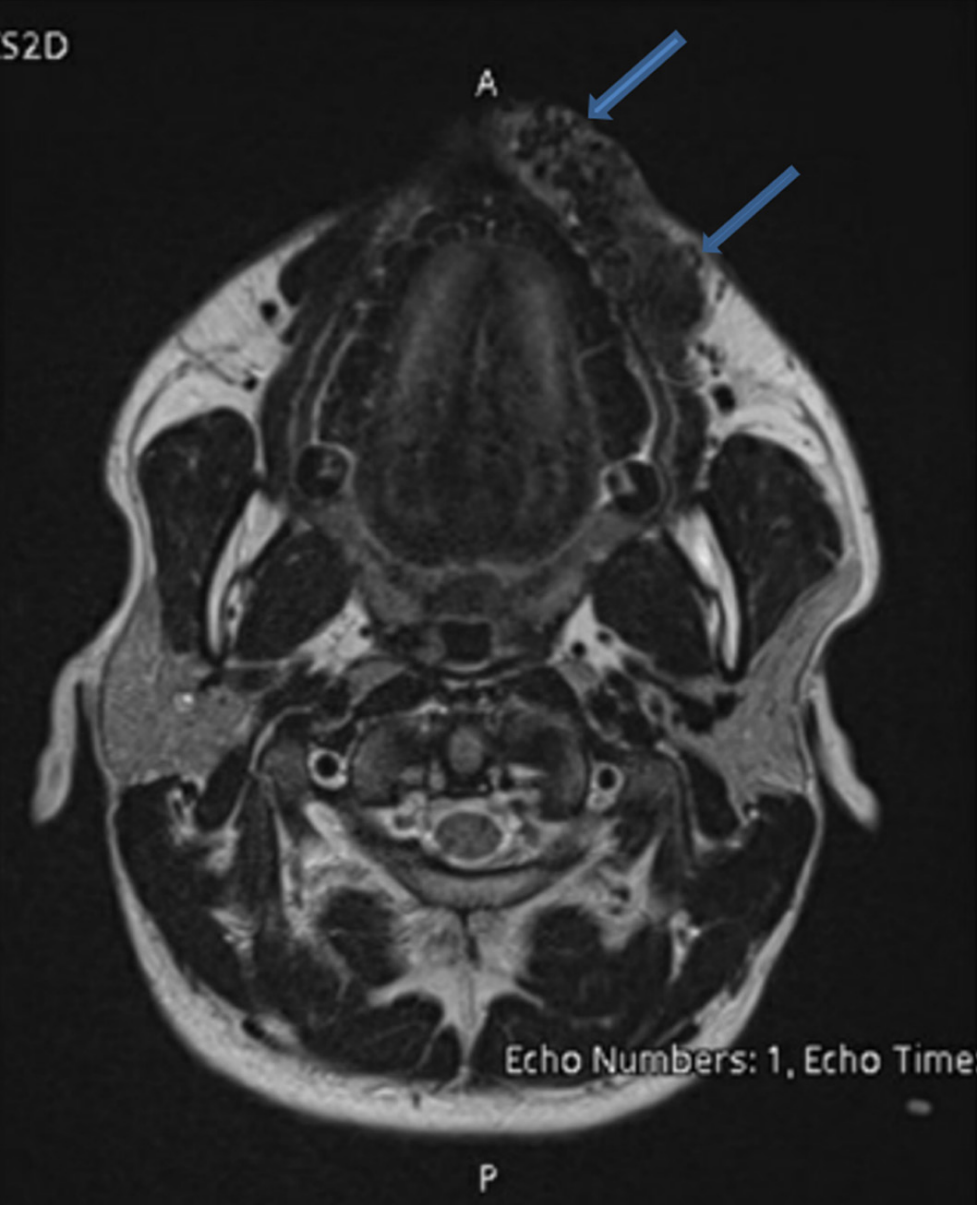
Magnetic resonance imaging (MR) of the head and neck (axial plane, T2‐weighted image) showing a diffuse linear‐tubular signal void areas located in the soft tissue of the lip at the level of the alveolar process (blue arrows).
